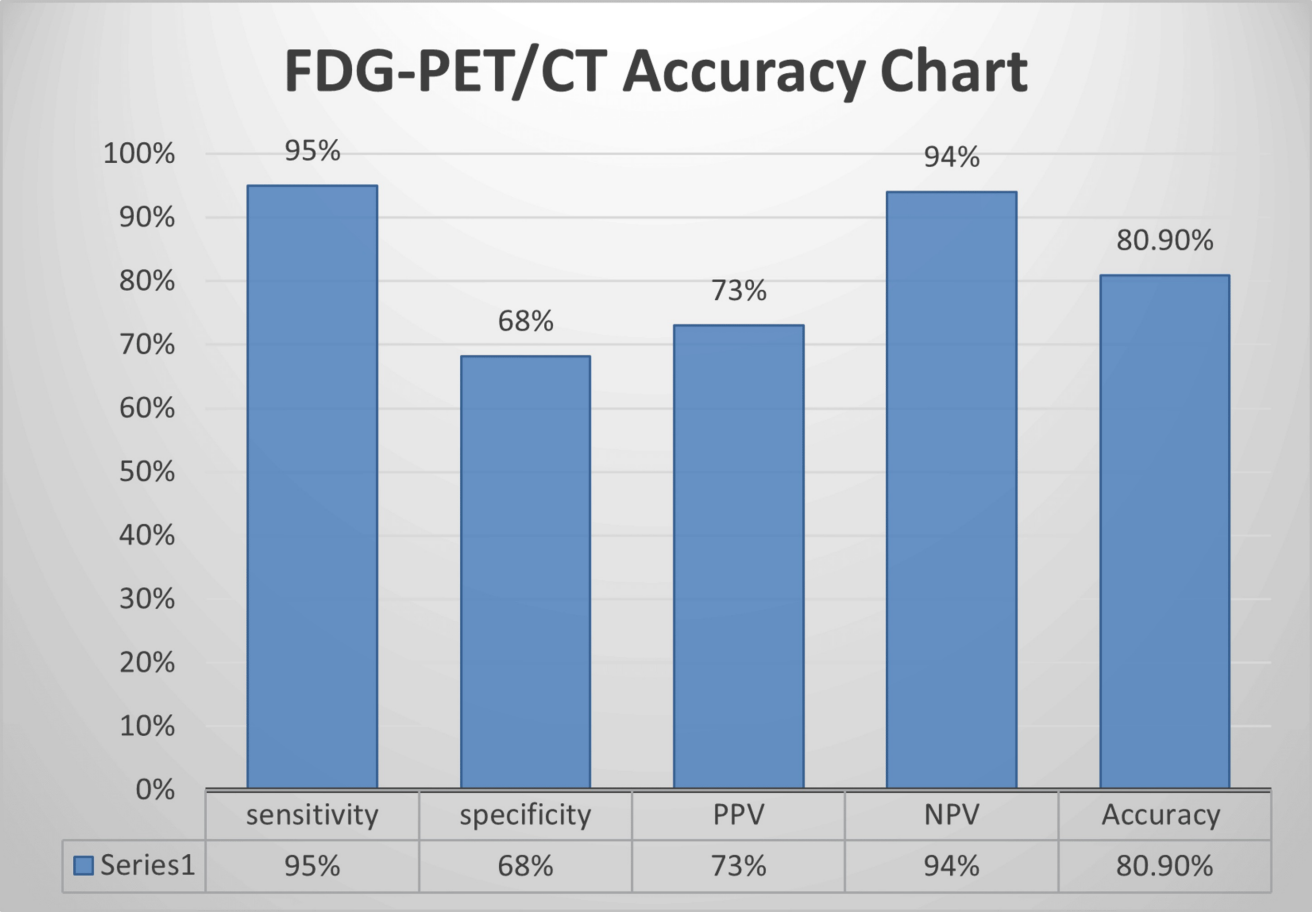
FDG-PET/CT accuracy chart depicting calculated specificity and sensitivity rates based on the results of the study FDG-PET/CT: 18F-fluorodeoxyglucose positron emission tomography/computed tomography
